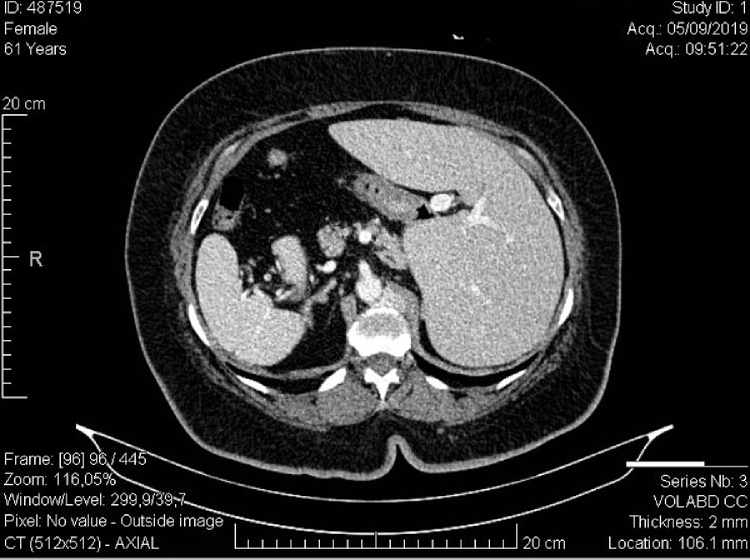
CT scan of the upper abdomen of the patient.
